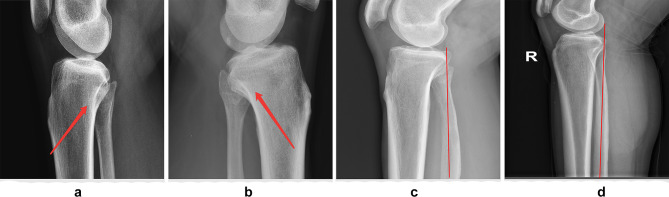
Visualization of occlusion and variation of the fibular head. a and b show occlusion of the fibular head, making the location of key point C ambiguous and resulting in inaccurate LAT overlap ratios. In c and d, the fibular head is bent due to variations, where the red line indicates the orientation of the fibula. The curved fibular head distorts the calculations of Sc, Sd, and Se, resulting in inaccurate LAT overlap ratios. Due to occlusion and variation, the fibular head overlap ratio is generally less consistent on LAT knee radiographs than on AP knee radiographs
